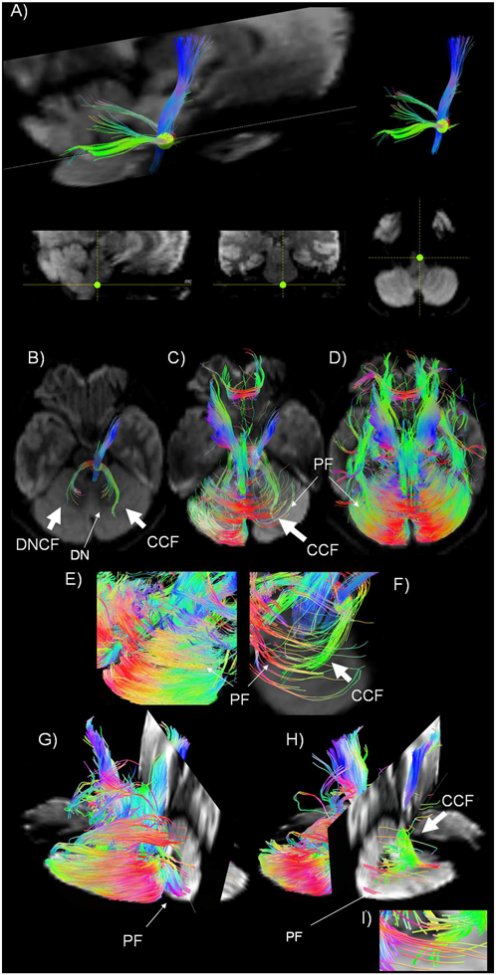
Olivary-cerebellar cortex connections and olivary-dentate-olivary loop. A) Sagittal tractography view, in a DWI image background on the left, showing the connections between (i) the Inferior olivary nucleus (green region of interest -ROI) and the cerebellar cortex (light bright green fiber trajectories) and (ii) the Inferior olivary nucleus and the Dentate nucleus (dark green fiber trajectories). The ROI in the Inferior olive is shown in the sagittal, coronal, and axial planes in the images at bottom (from left to right). B), C) and D) Axial tractography view, in a DWI background, showing: B) the connections between (i) the Inferior olivary nucleus (green ROI) and the dentate nucleus (DN) through Dentate nucleus climbing fibers (DNCF) and (ii) the connections between the inferior olivary nucleus (green ROI) and the cortex through cortical climbing fibers (CCF); C) the intersection between the CCF and parallel fibers (PF) from the granule cell axons. D) PF in the cerebellar cortex. E) and F) Tractography magnification of an axial view of the cerebellar cortex, in DWI background. PF and on the left, PF intersecting CCF. G), H), I) 3D tractography view, in a DWI background, showing: G) some PF traversing the cerebellar cortex. H) PF crossing CF that are oriented perpendicular to them. I) Higher magnification of H).
